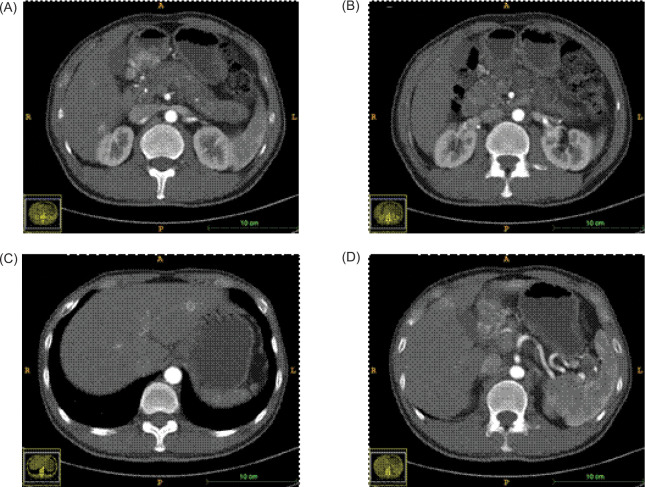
CT scanning during the first diagnosis (arterial phase). (A) lesion in the gastric antrum; (B) lesion in the left renal hilus; (C, D) lesions in the liver.
